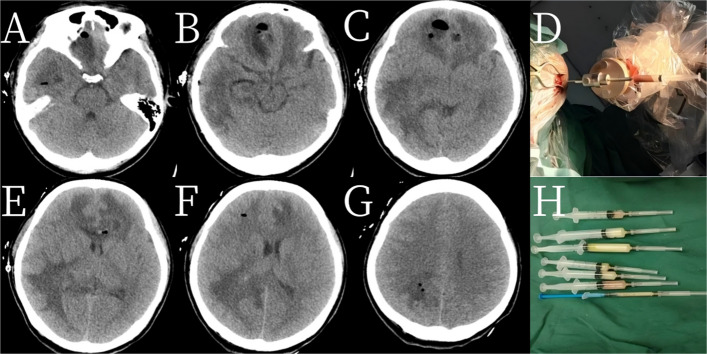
A–C, E–G Postoperative CT scans demonstrating successful aspiration of the brain abscesses with restoration of midline structures and no evidence of hemorrhage. D Intraoperative image of a stereotactic puncture. H Intraoperative aspiration of the purulent material
